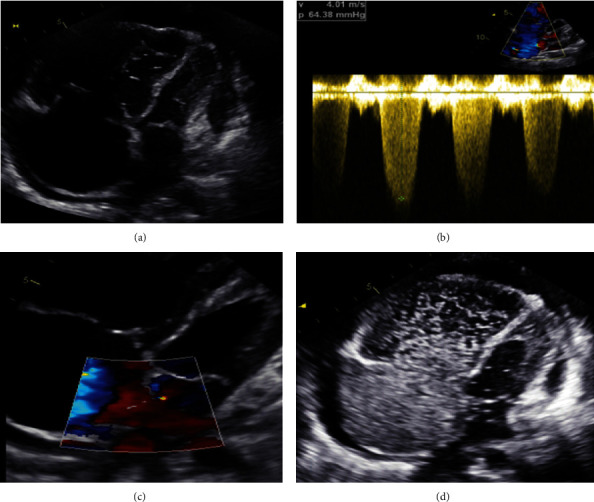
Transthoracic echocardiography revealed severe dilatation and systolic dysfunction of the right ventricle while normal left ventricle and moderate pericardial effusion (a). There was severe TR with an estimated PASP >80 mmHg and TRV 4.01 m/s (b). The right atrium was severely dilated with a small atrial septal defect (c). TEE revealed a positive agitated saline test confirming interatrial shunting (d).
